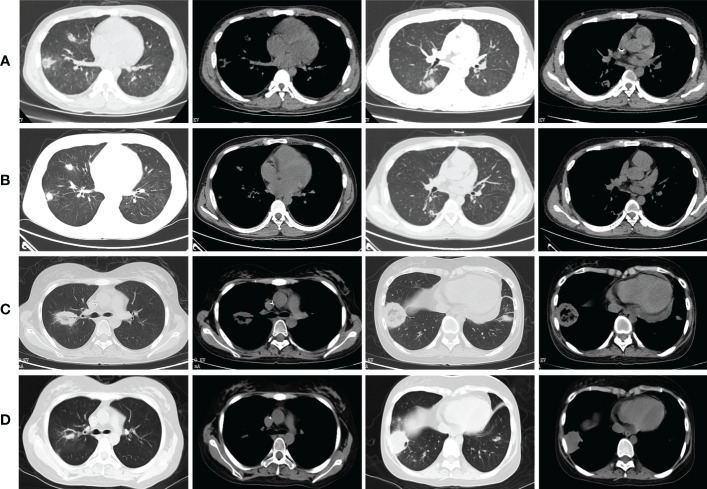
Findings of chest CT scans before and after ABCD treatment in cases 3 and 4. (A) Before ABCD treatment, multiple nodules and reversed halo signs were seen in both lungs; (B) After 6 weeks of ABCD treatment, both lung lesions were significantly reduced; (C) Before ABCD treatment, reversed halo signs were observed in the upper and lower lobes of the right lung; (D) After 6 weeks of ABCD treatment, the lesions were significantly reduced, and cavity was formed in the upper lobe of the right lung, while low-density necrosis and small cavity was found in the lesions in the lower lobe of the right lung.
